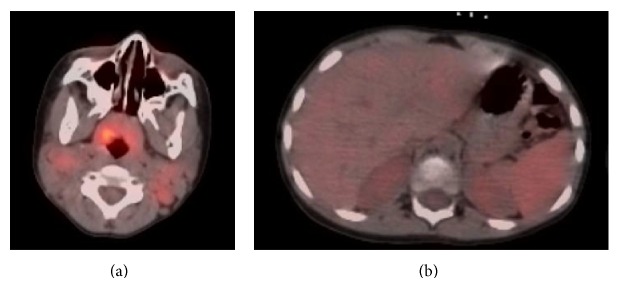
PET/CT demonstrates increased tracer uptake within bilateral cervical lymph nodes and the nasopharyngeal region (often normal in children). Slightly increased uptake in the spleen is seen in the image on the right with no apparent focal lesion.
